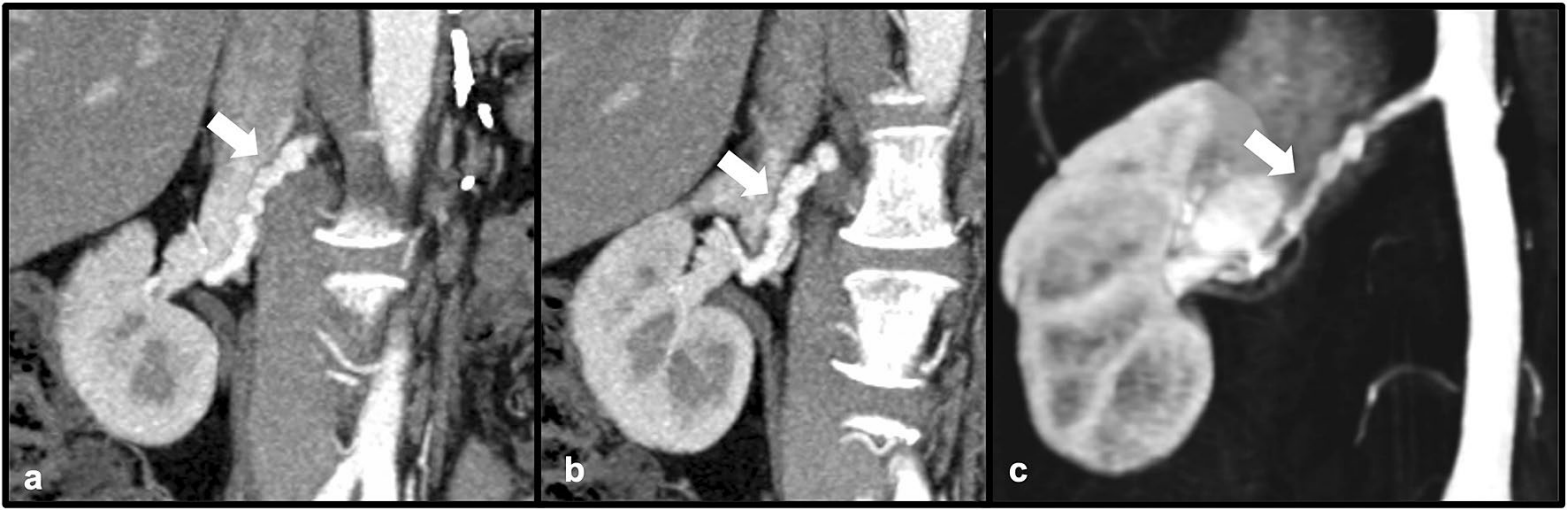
52-year-old male with oliguria and abnormal renal function. Coronal contrast-enhanced CT (a and b) demonstrate beading and irregularity of the right main renal artery (arrows). Coronal post-contrast MRI (c) also demonstrates beading and irregularity of the right renal artery (arrow) consistent with FMD
